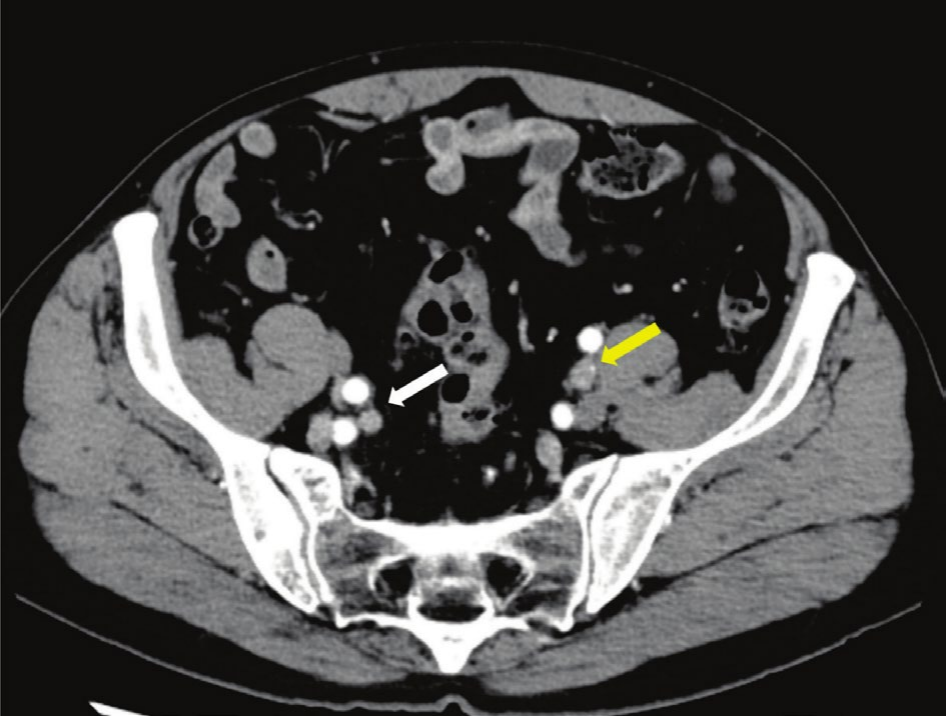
Contrast‐enhanced CT. Contrast‐enhanced CT revealed bilateral enlargement of the external iliac lymph nodes (white arrow, right external iliac lymph node; yellow arrow, left external iliac lymph node).
